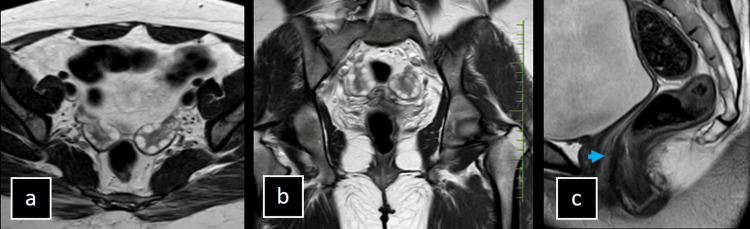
(a-c) Imaging of Mayer-Rokitansky-Küster-Hauser (MRKH) Syndrome in a Patient With Primary Amenorrhea. MRI of a 17-year-old female presenting with primary amenorrhea reveals non-visualization of the uterus, cervix, and upper two-thirds of the vagina, with a hypoplastic lower segment of the vagina. The bilateral ovaries appear normal. These findings are indicative of MRKH syndrome.
